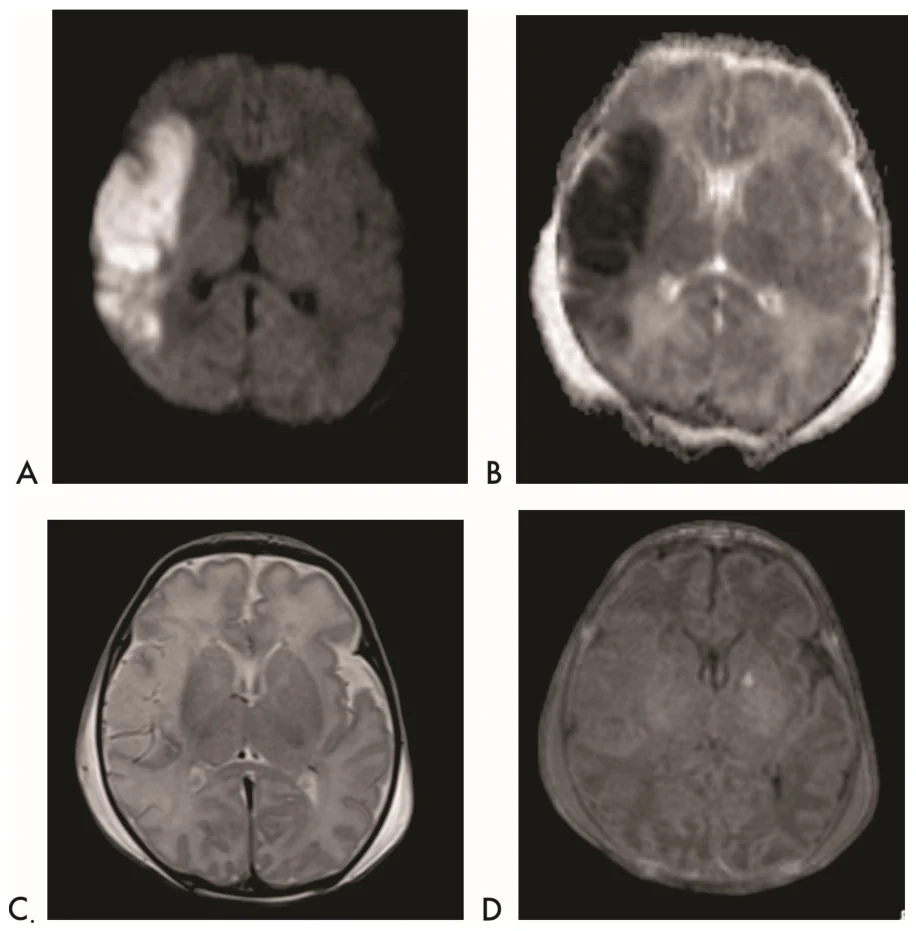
Case: MRI day 4. (A,B) diffusion-weighted images (A. SB100 and B. ADC) demonstrating right middle cerebral artery territory infarct. (C) T2 image demonstrating corresponding edema. (D) T1 image with hyperintensity in the left basal ganglia, most consistent with hemorrhage.
